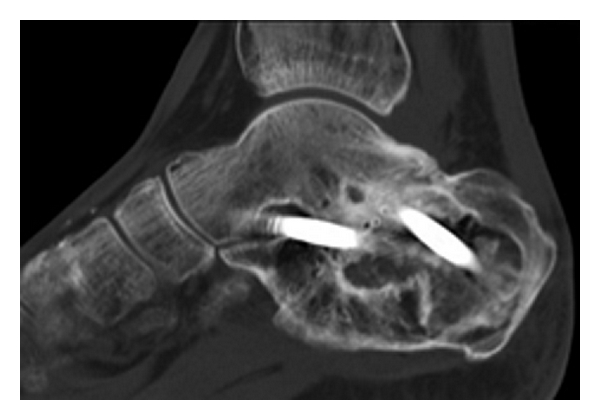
Sagittal computed tomography scan obtained 6 months postoperatively showing union of the posterior subtalar joint and incorportation of the bone grafts. Two large cannulated, 7.5-mm screws were used. The horizontally placed screw fixed the talar head, the other screw the talar body.
